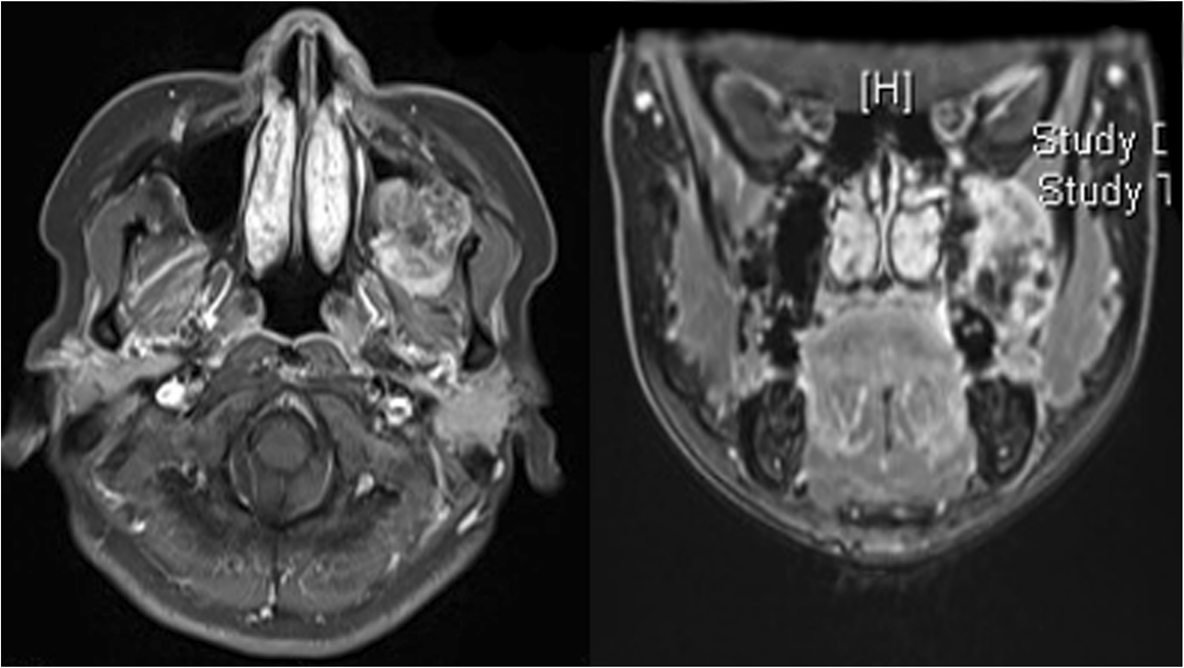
Magnetic resonance imaging showing diffuse mass with the contrast effect existing mainly in the pterygopalatine fossa. The contrast-enhanced areas correspond with the radiolucent area on computed tomography
